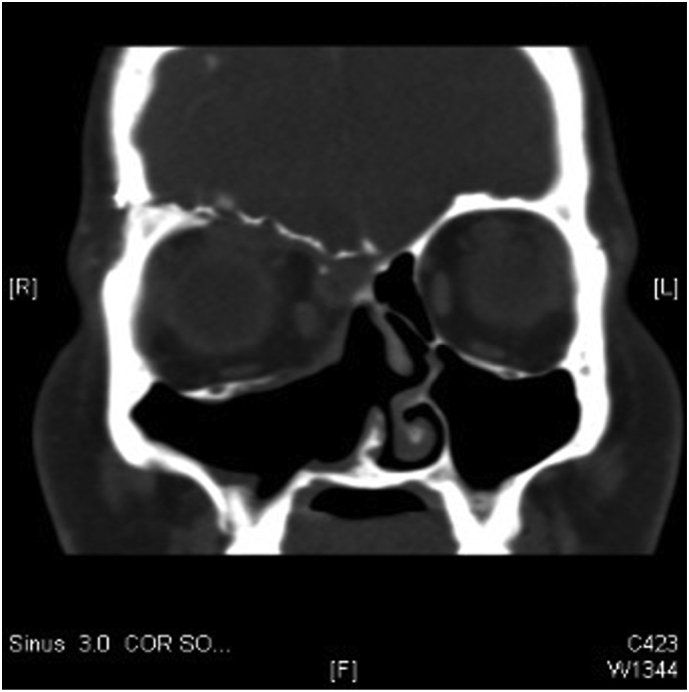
Post-operative paranasal coronal CT scan.
